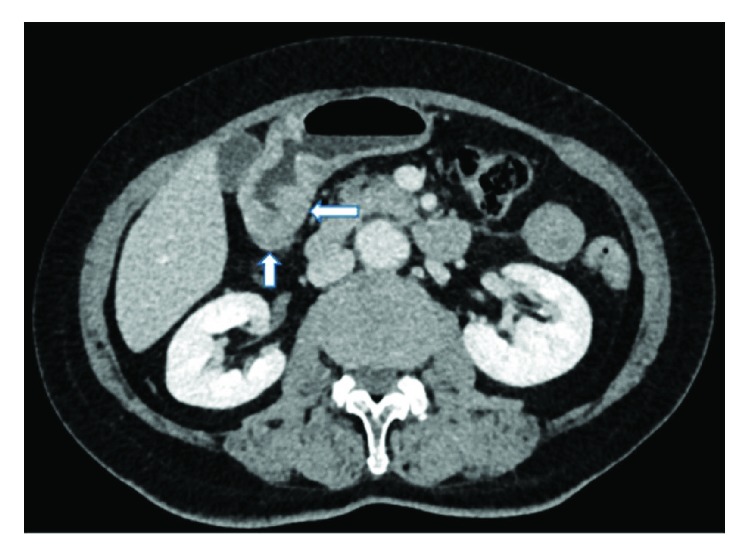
CT image obtained in a 75-year-old woman. The serosal surface is smooth around the lower 1/3of the stomach.
